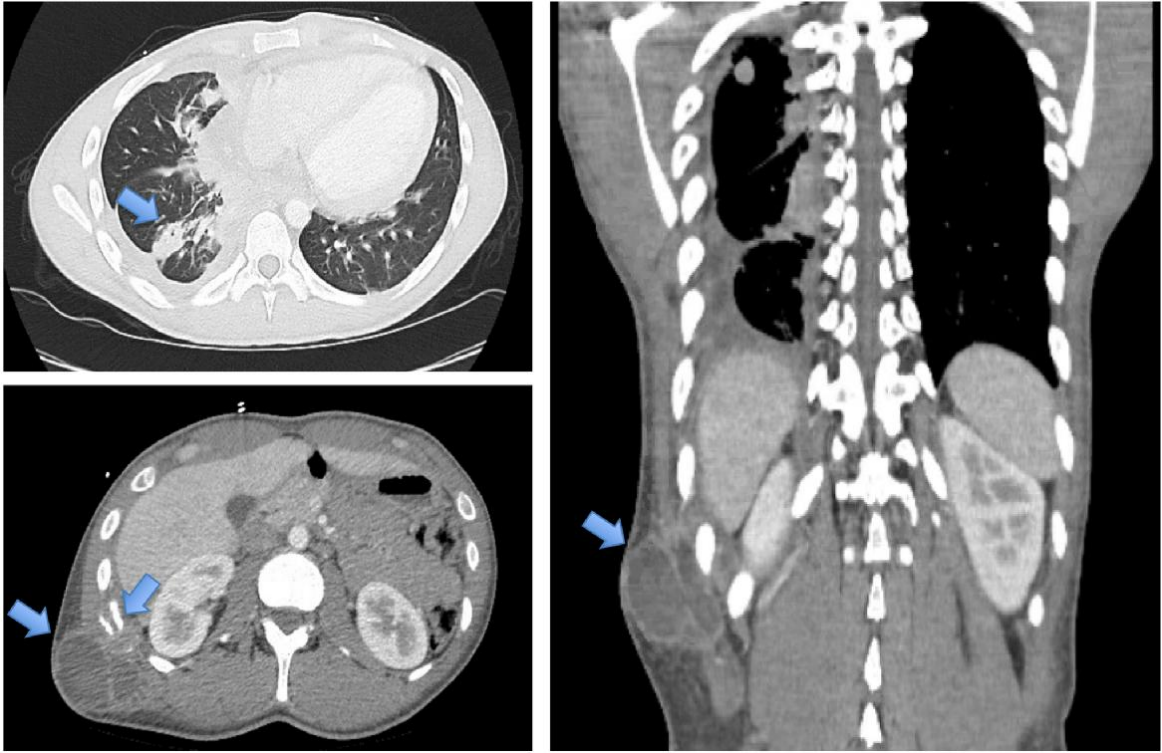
Chest and abdomen CT scan, demonstrating pulmonary, osseous, and cutaneous involvement. Abbreviation: CT, computed tomography.
